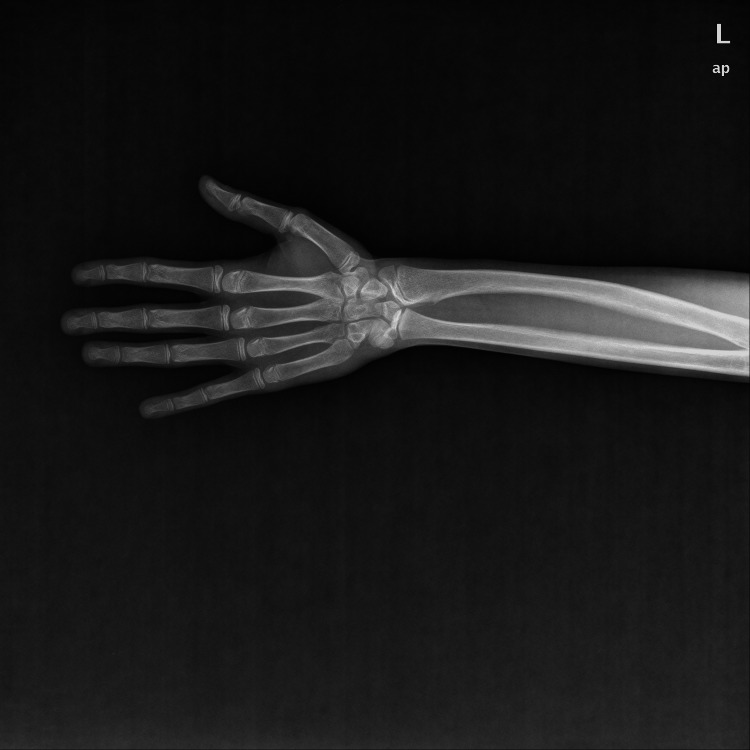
Left wrist X-ray showing bone modeling disease.
